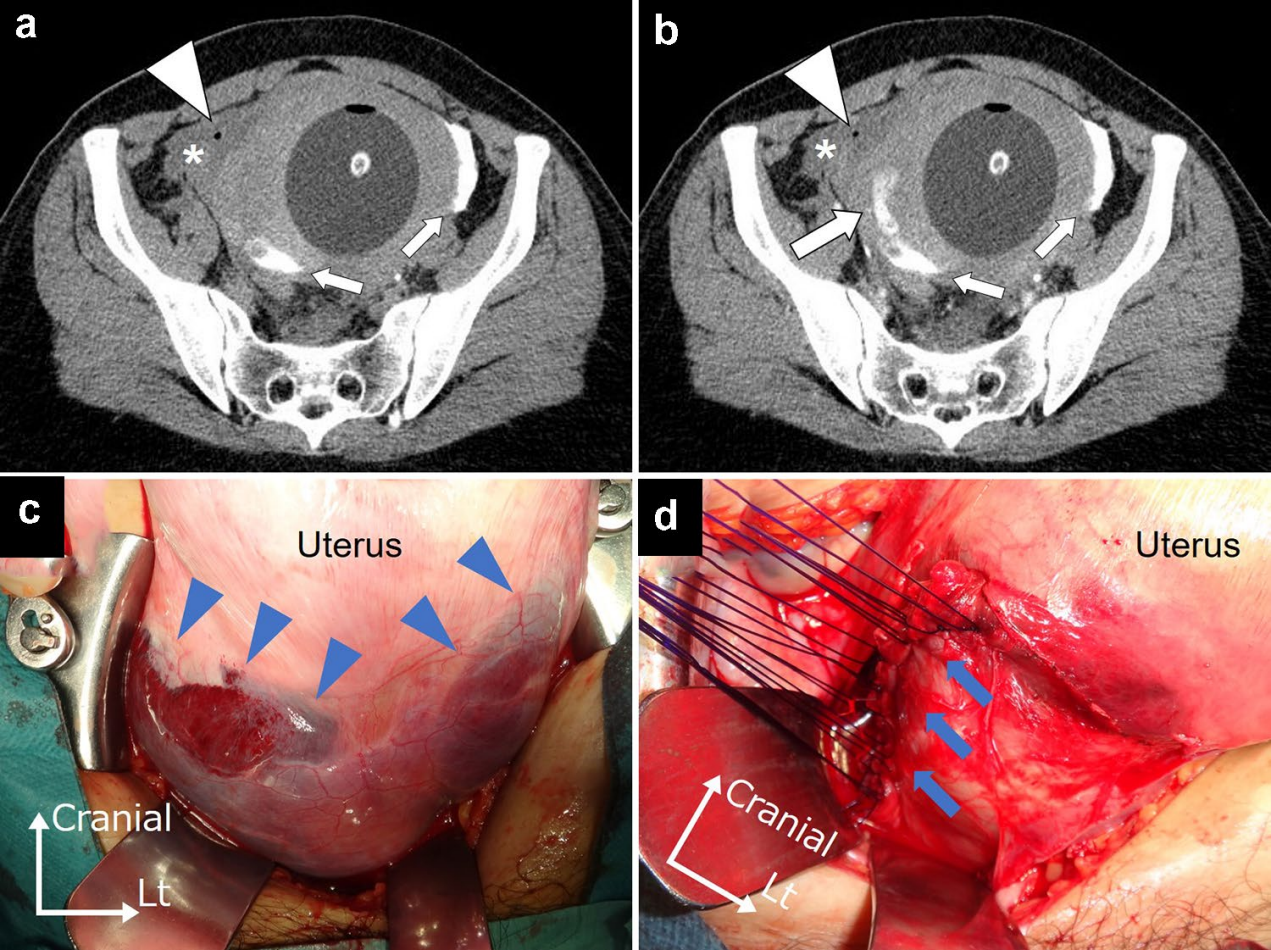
Patient 1: a unenhanced and b contrast-enhanced computed tomography images obtained during the arterial phase demonstrating extravasation (large arrow). The contrast medium used during uterine artery embolization is visible (small arrows). A hematoma (asterisk) and free air (arrowhead) can be seen in the broad ligament. An intrauterine balloon tamponade device was placed. c Hematomas spreading in the broad ligaments (blue arrowheads). d The broad ligament was incised, and a 10 cm laceration on the right side of the lower uterus was sutured (blue arrows)
